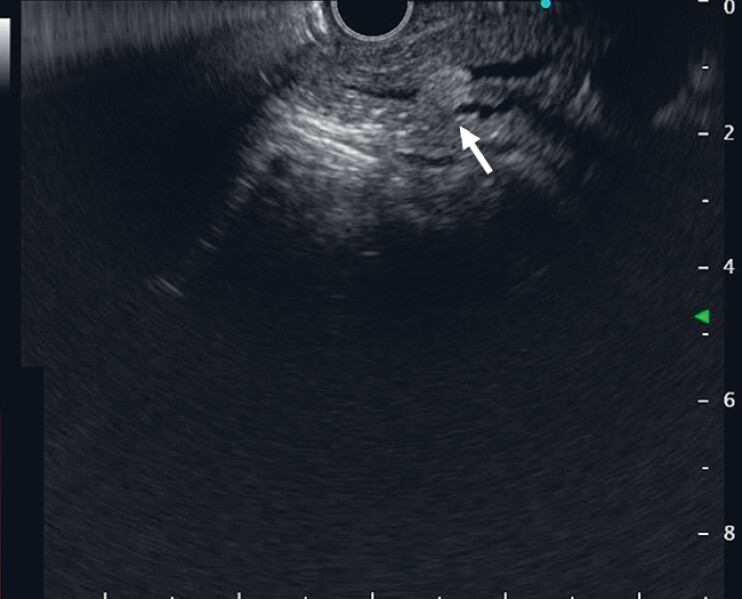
Endoscopic ultrasound showed the calcified stone (arrow) impacted in the pancreatic duct.
